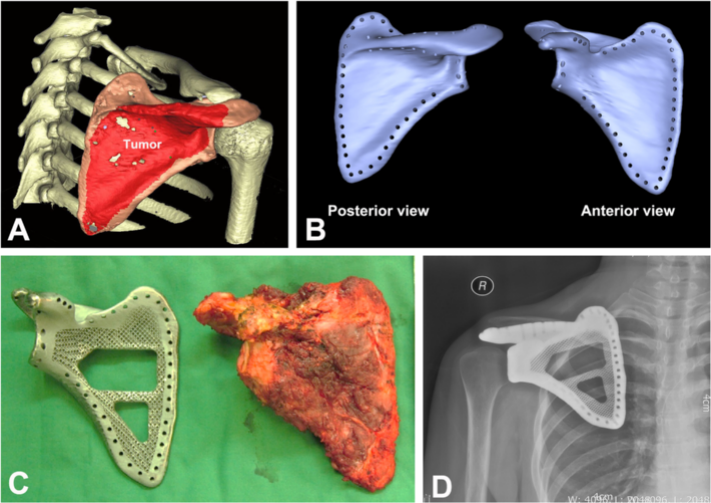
a Reconstructed 3-D scapular tumor model. b The virtual 3-D model of scapular prosthesis. c The size of excised tumor and prosthesis was well matched. d X-ray showed a proper shoulder articulation at 21 months postoperatively
